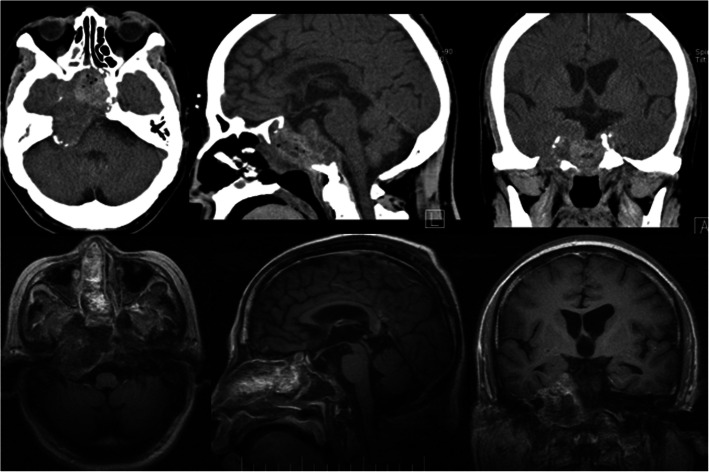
The preoperative CT (computed tomography) and postoperative MRI (magnetic resonance imaging) of a recurrent chordoma: the patient, male, 59 years old, chief complaint: trans-sphenoidal chordoma resection and radiotherapy for 6 years, right hearing loss accompanied by peripheral facial paralysis for 3 months. The tumor of sphenoid sinus, ethmoid sinus, and petrous apex region was totally resected via the endoscopic transethmoid–pterygoid process–sphenoid sinus approach and middle approach extended to petrous apex region
